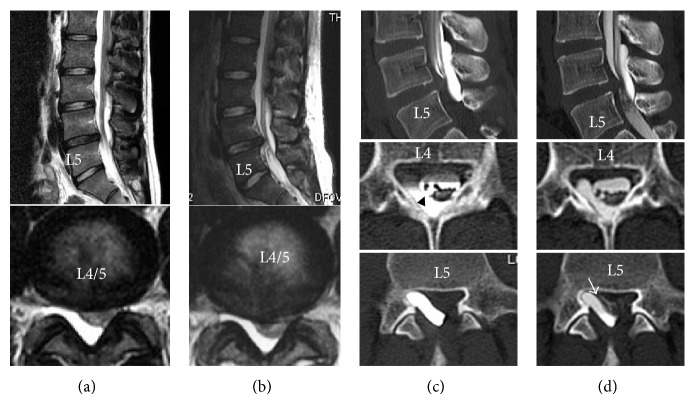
Magnetic resonance (MR) images immediately after injury show a dorsally located cystic lesion extending from L3 to L5 and disc herniation at the L4-L5 level (a). Preoperative magnetic resonance images show progression of herniation and compression of the dural sac (b). Computerized tomography (CT) myelogram shows the communication site at the L4 level ((c), arrowhead). Compression of the cauda equina and blockage of CSF at the L4-L5 level are also shown. CT myelogram obtained 2 h later demonstrates the L5 root sandwiched between the cyst and herniation ((d), arrow).
